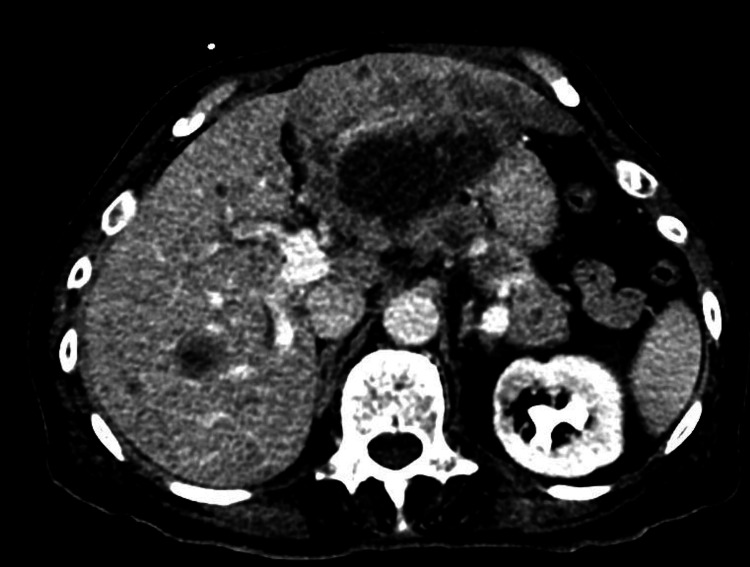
Axial CT images of the patient’s liver showing multiple metastases and the initial planned outpatient site of biopsy prior to admission CT = computed tomography
